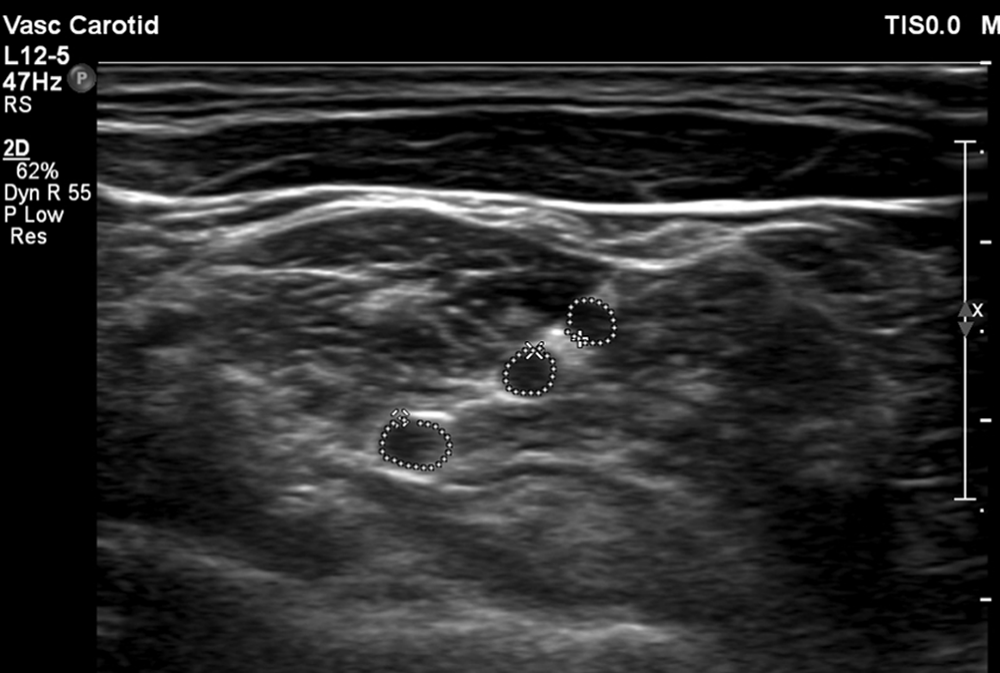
Short-axis sonographic image of the C5-C7 nerve root cross-sectional areas obtained with tracer measurements.
